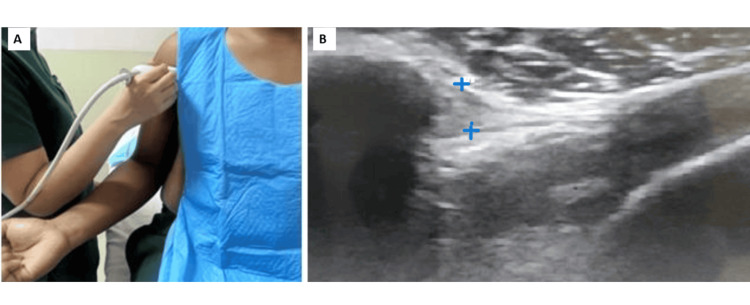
Measurement of the coracohumeral ligament thickness by ultrasonography A) Position of the probe; B) Ultrasound image
